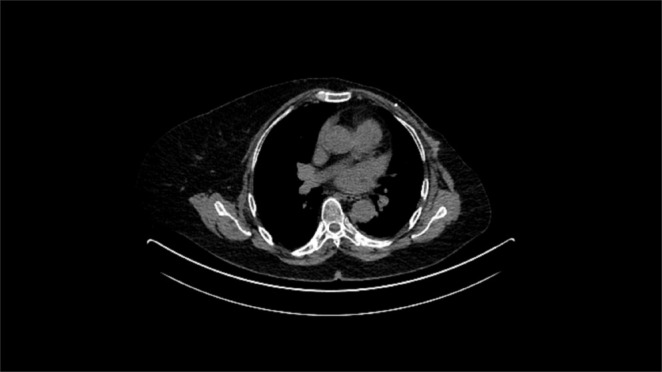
Last control CT evaluation in May 2024 shows no signs of disease.
